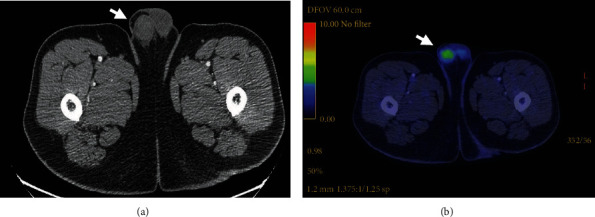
FDG-PET/CT scanning revealed a testicular mass, with maximal SUV of 6.9 (arrow): (a) transverse image and (b) CT of the testicular mass.
